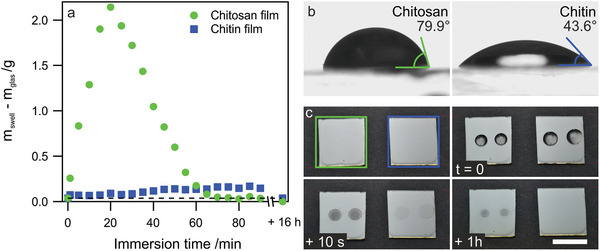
a) Mass change of CS and CT films after consecutive water immersion for 90 min and after complete drying. b) The water contact angle of CS and CT film was determined in a sessile drop experiment. The presented values are mean ± SD. c) Photographs of CS and CT films before and at different times after water droplet exposure. The scale bar is 2 cm.
